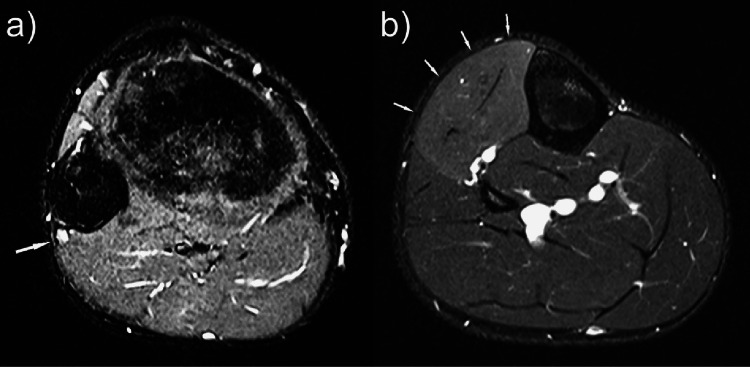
(a) Axial slice of an MRI STIR sequence of patient 1’s right knee demonstrating oedema of the common peroneal nerve as it passes around the fibula head. (b) Axial slice of an MRI STIR sequence of the right calf demonstrating oedema of the anterior compartment consistent with denervation. STIR, short tau inversion recovery
